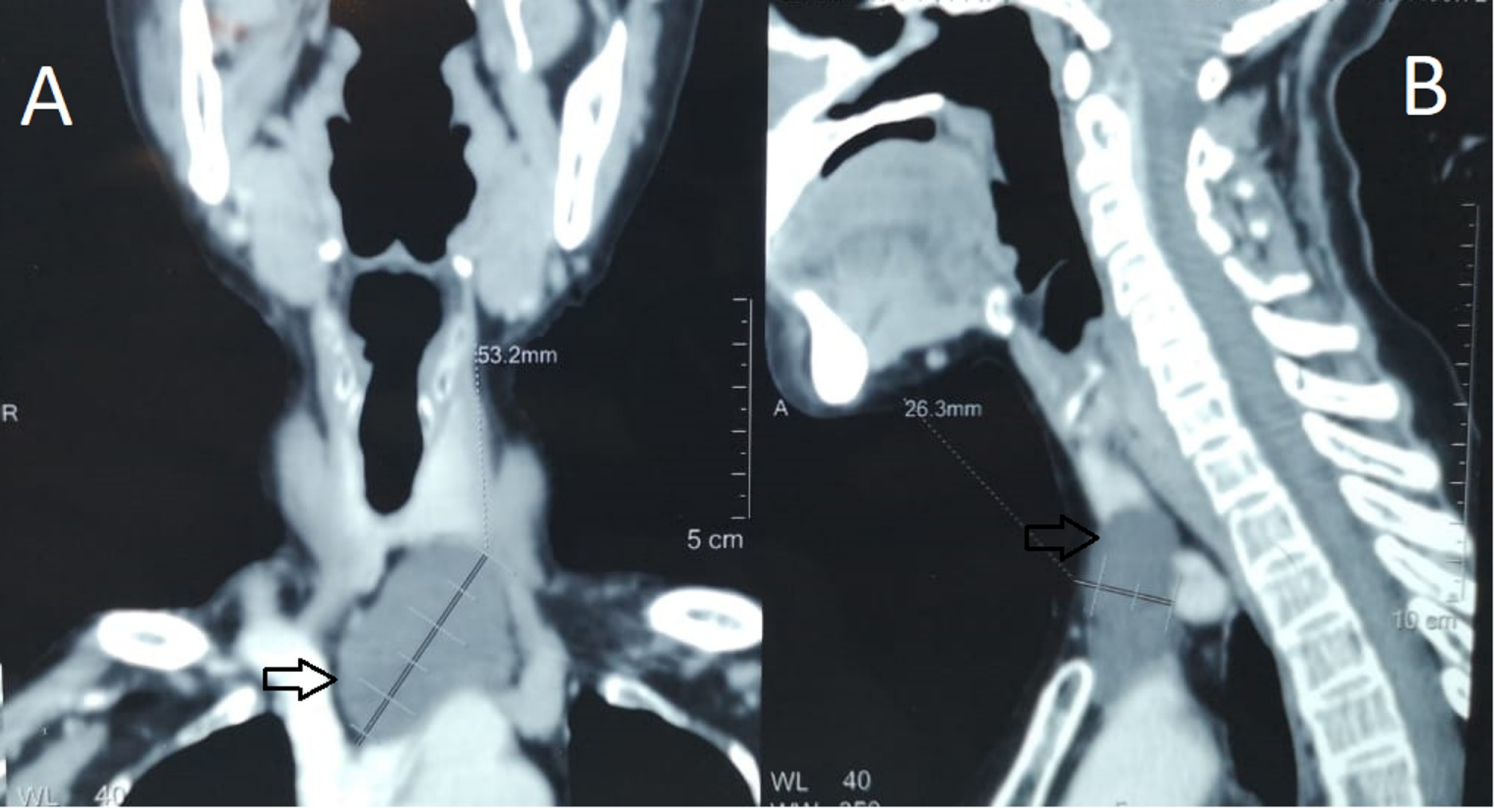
(A and B) Cervical computed tomography scan showing left-sided cervical cystic mass (black arrows) measuring 26x45x53 mm
